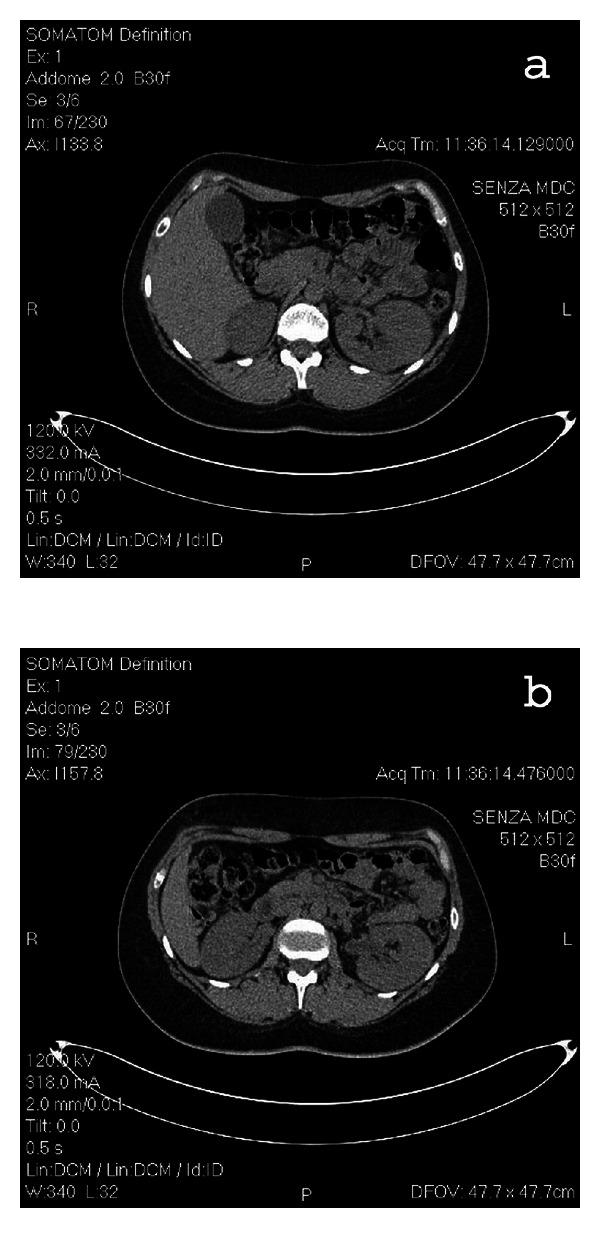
Uro-CT: (a) and (b) confirmation of the first degree hydronephrosis of the left kidney.
